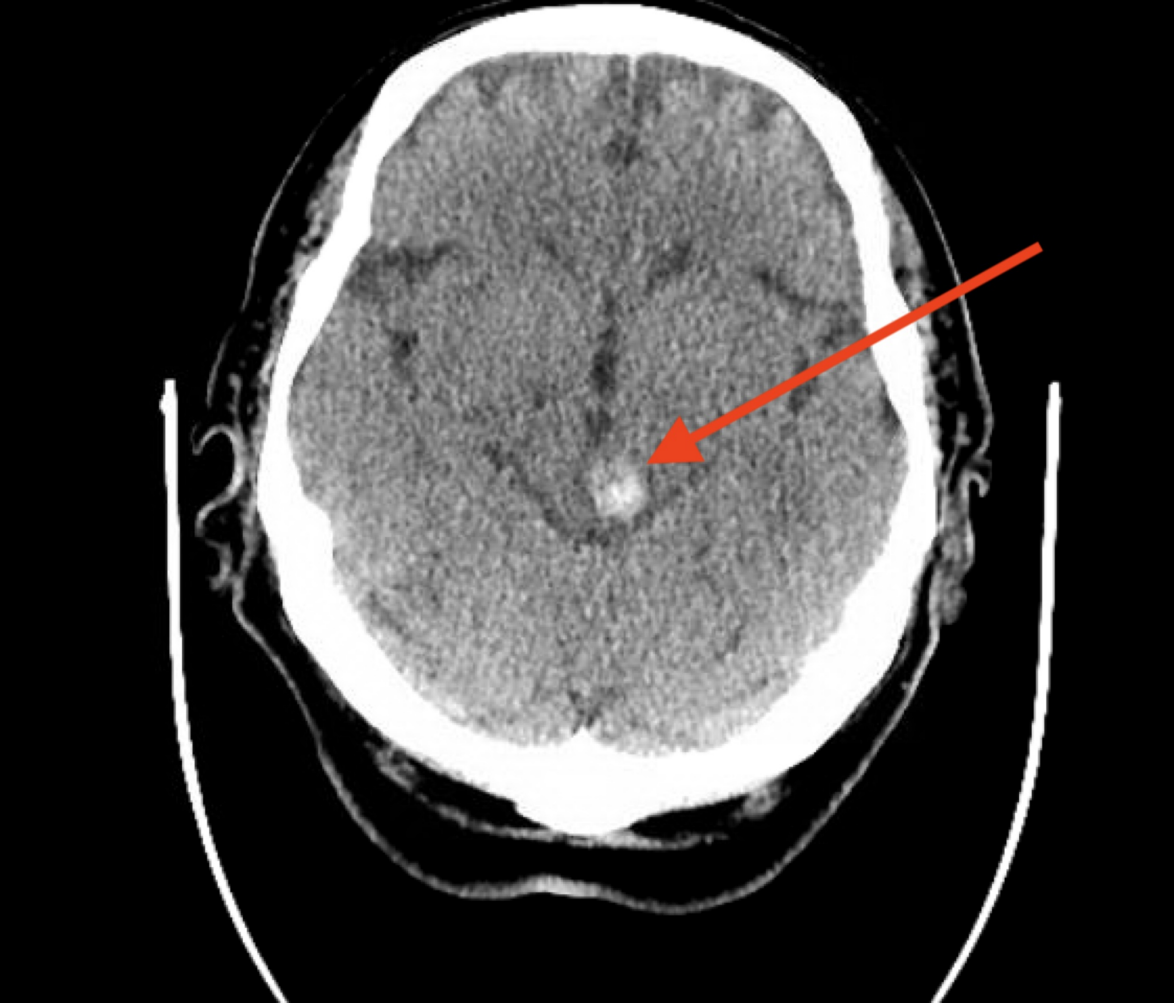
This is a CT without contrast at the time of presentation for neurologic deficits showing a rounded focus of varying hyperdensities suggesting hemorrhage of varying durations including acute and subacute hemorrhagic components.
